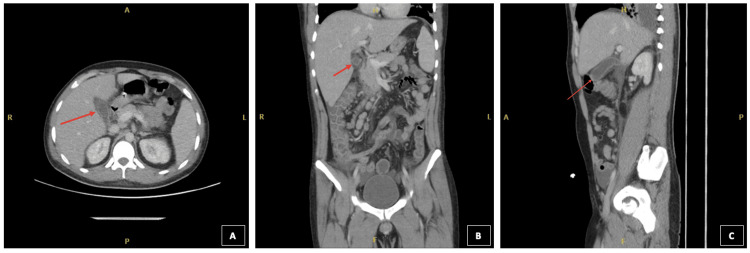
CT Thorax, Abdomen and Pelvis displaying a thick-walled gallbladder with surrounding pericholecystic fluid collection (as shown by red arrows) (A) Axial view; (B) Coronal view; (C) Sagittal view.
